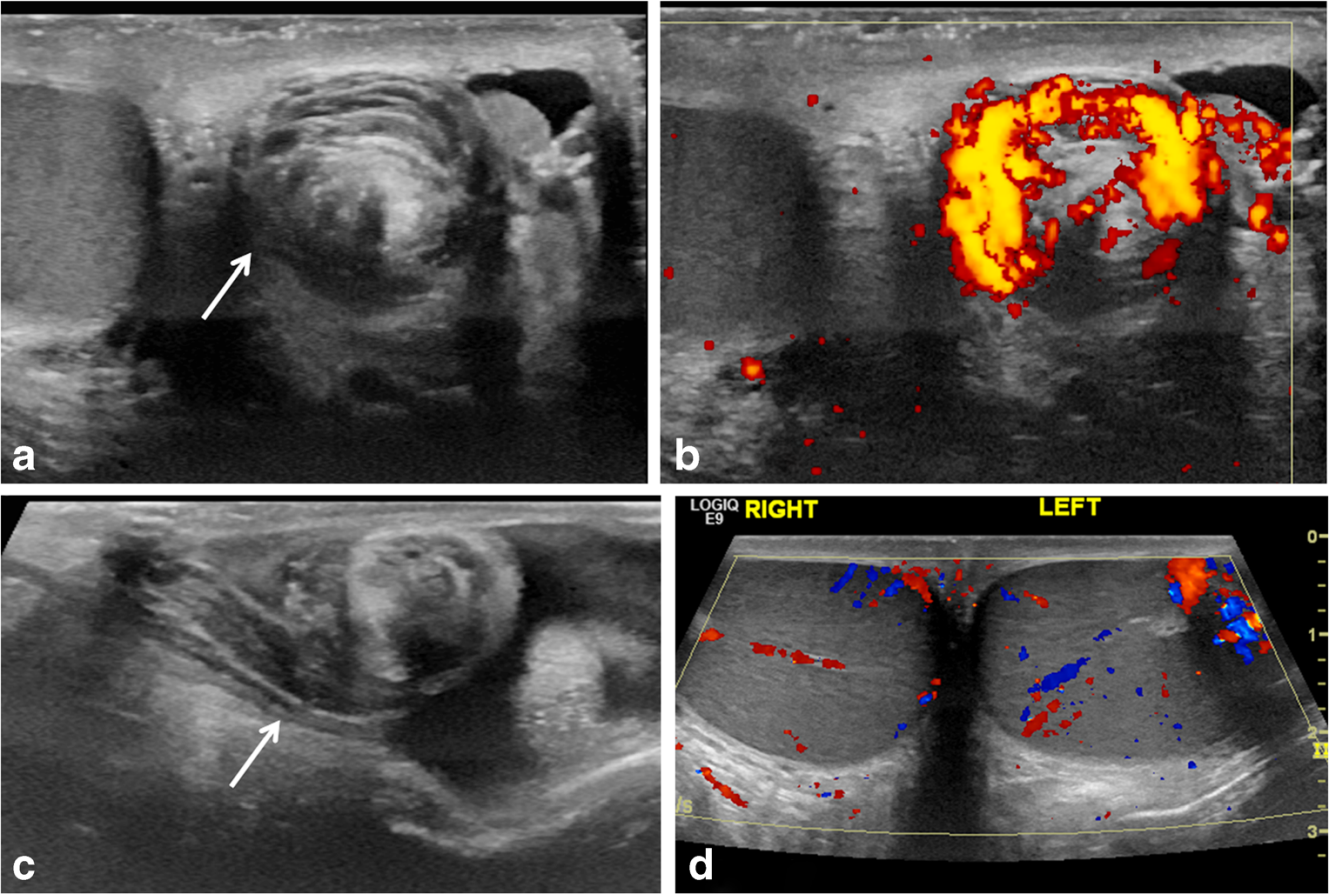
“Whirlpool sign” of spermatic cord. a Gray-scale transverse US image of upper left scrotal sac shows an eddy swirl (*arrow*) of the spermatic cord suggesting torsion of the cord. This 12-year-old boy woke with acute left testicular pain and experienced nausea and vomiting along with the pain. b Power Doppler US image of the same twisted cord shows concentric pattern of preserved flow in the vessels of the twisted cord. The flow in the left testis (not shown) was minimally decreased compared to the right side and bilateral bell clapper deformity was found during orchiopexy along with complete torsion of the left testis with 360° twist. c Gray-scale longitudinal US image of the left scrotum in a 13-year-old boy with 1 day of left-side pain shows abrupt spiral twisting of the spermatic cord (*arrow*) at the external inguinal ring, creating a whirlpool sign. d Color Doppler transverse image of the testes in the same boy as in (c) shows preserved and symmetrical flow bilaterally. After manual detorsion in the emergency room, he underwent orchiopexy and was diagnosed with intermittent torsion
